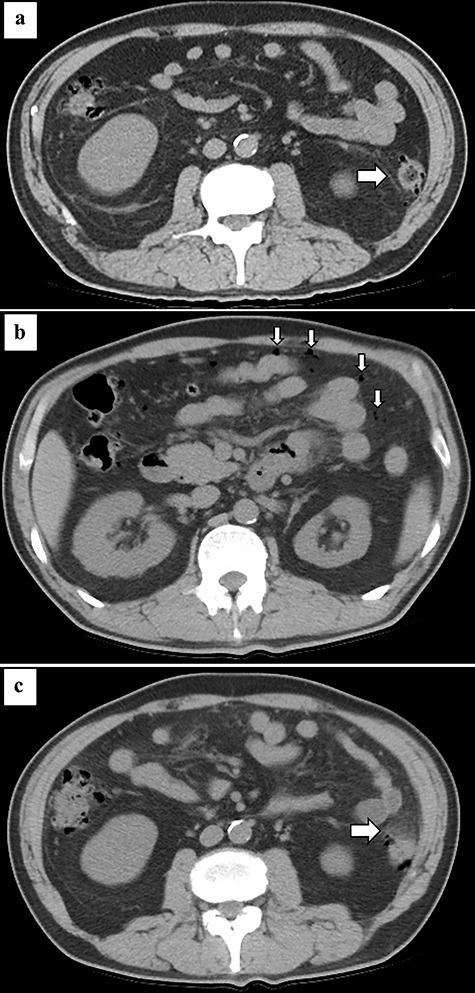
Abdominal CT at the onset of COVID-19 showing diverticulum of the descending colon (arrow) (a). Abdominal CT at the onset of pan-peritonitis showing free air in the abdominal cavity (arrow) (b) and increased fat density surrounding the descending colon (arrow) (c).
